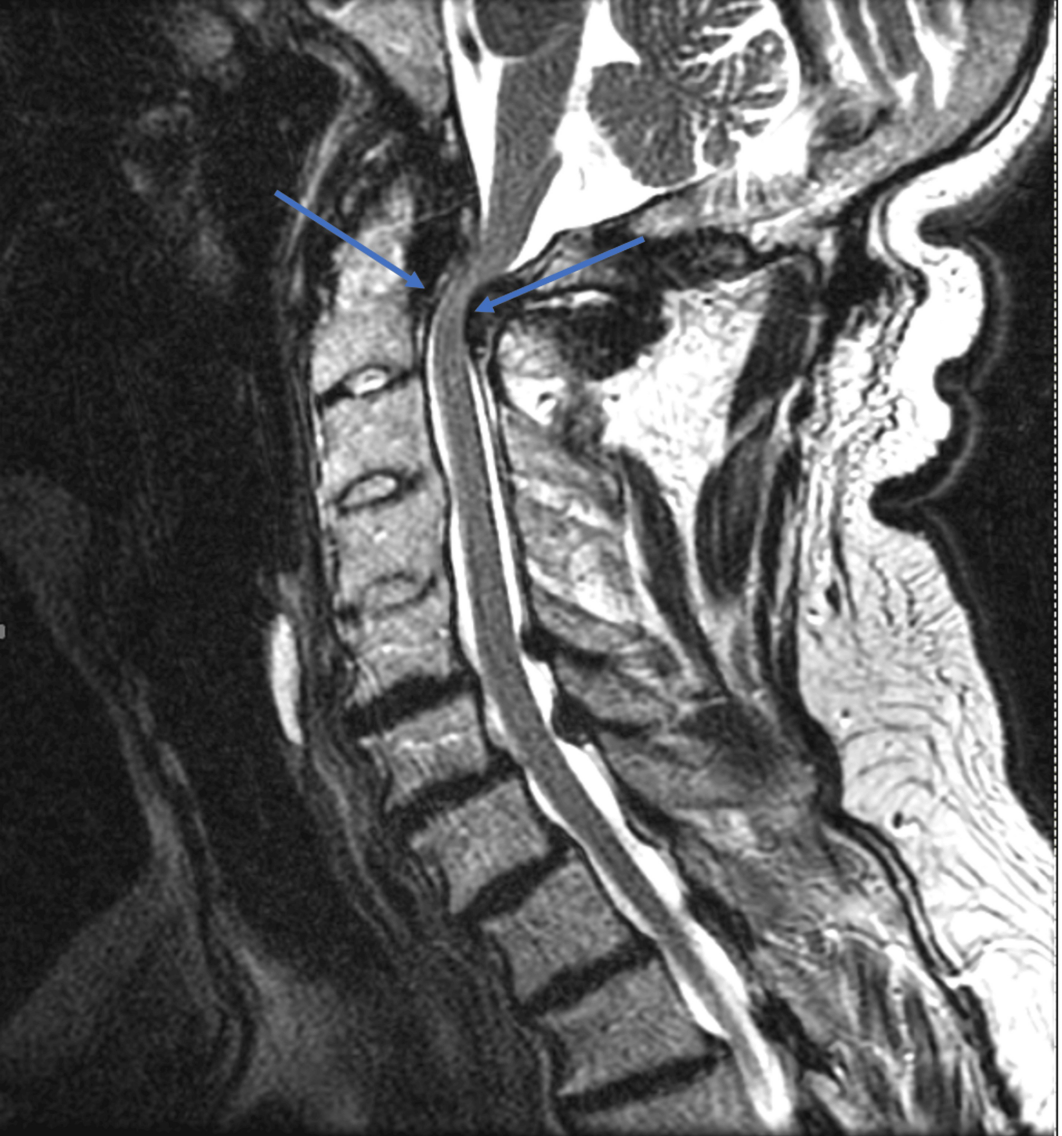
Cervical Spine MRI Sagittal T2-weighted MRI of the cervical spine. Arrows designate areas of severe stenosis at the C1-2 junction with both anterior and posterior compression.
